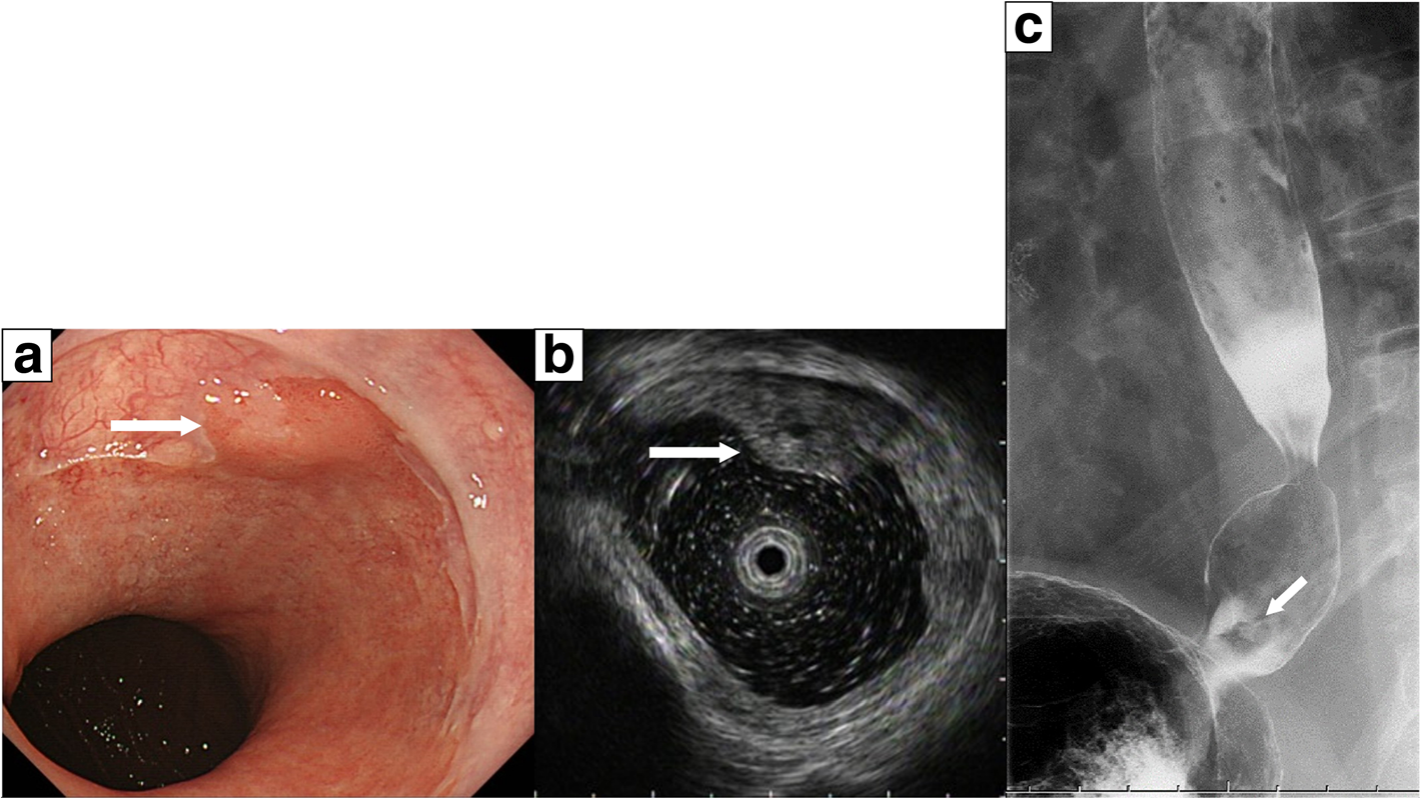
Images obtained before surgery. a Endoscopy shows a sliding hiatal hernia and an approximately 10 mm elevated mass at the esophagogastric junction. b Endoscopic ultrasonography shows a mass having mixed echogenicity in the esophageal wall, with partial invasion of the submucosal layer. c Upper gastrointestinal imaging shows an elevated lesion at the esophagogastric junction
